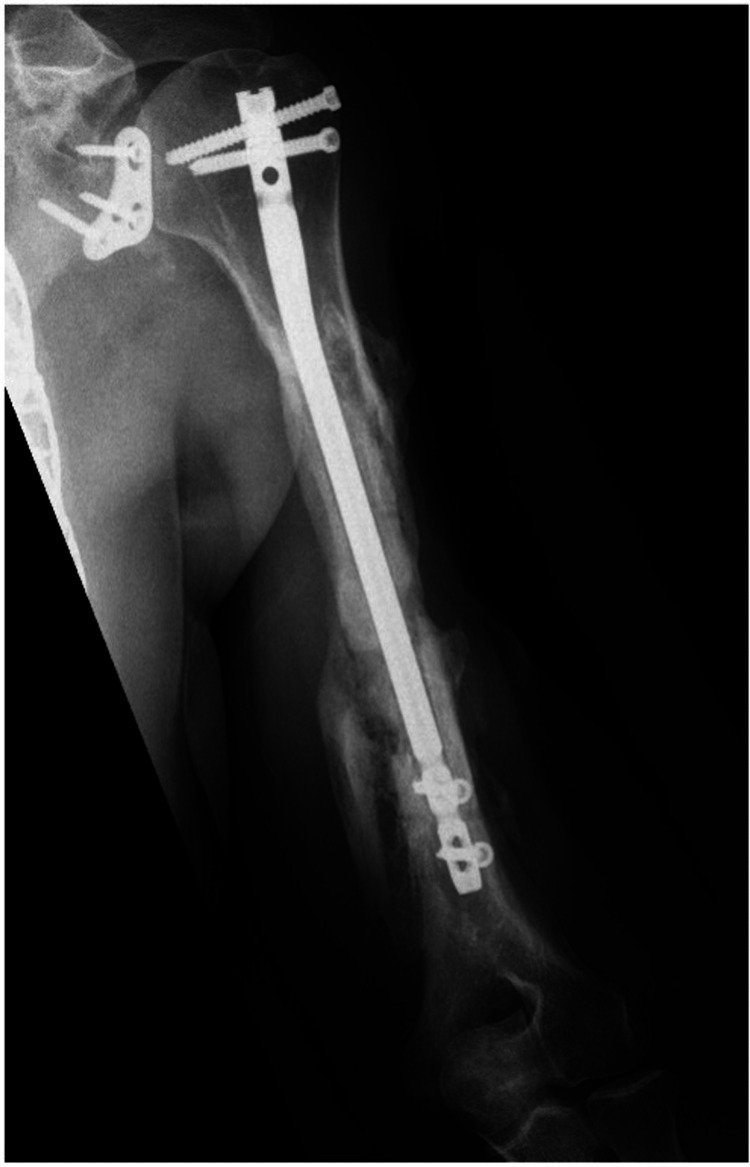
Anterior/posterior humerus radiograph demonstrating a healed comminuted fracture at one year follow up.
